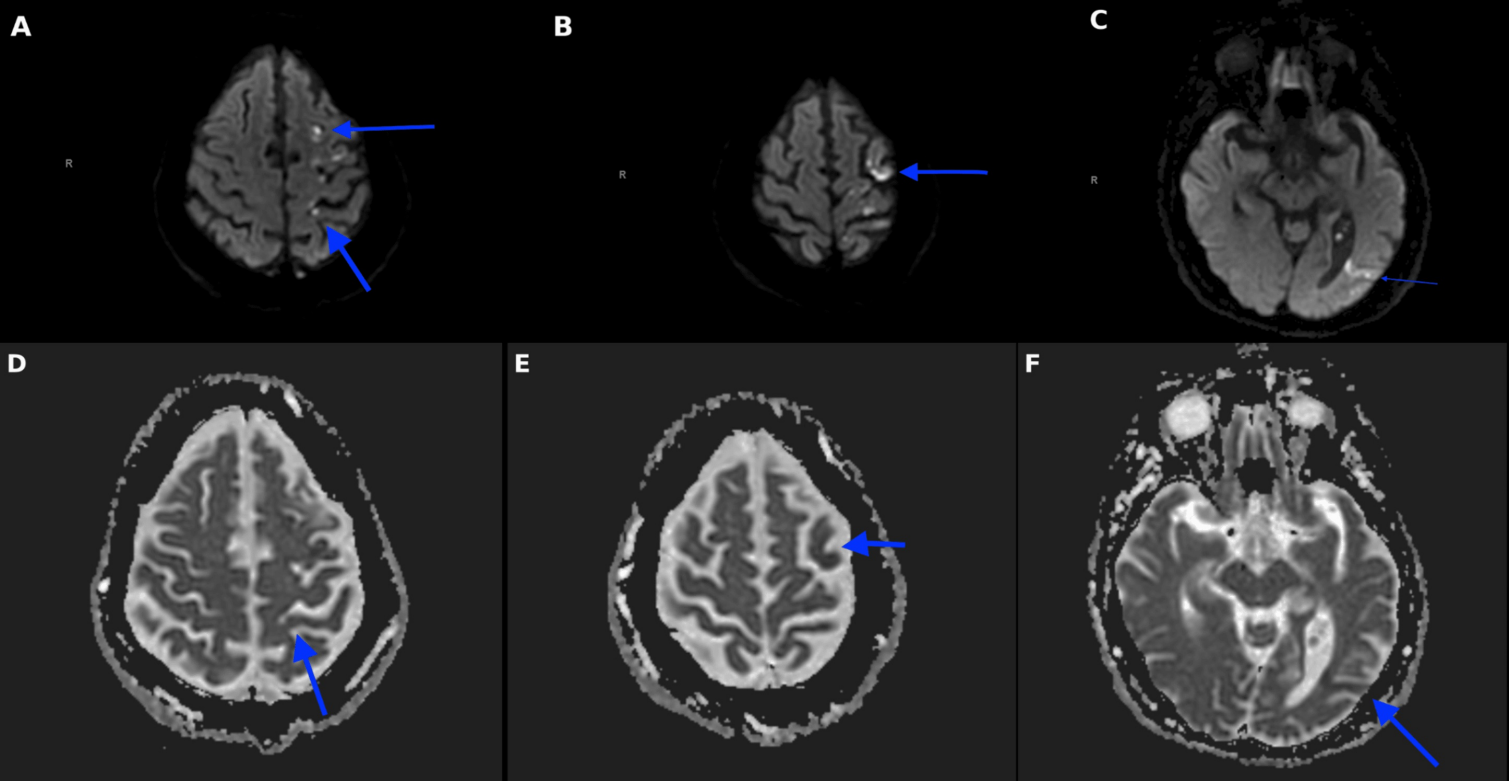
Case 1: (A-F) Numerous areas of restricted diffusion involving the left frontal lobe, parietal lobe, and left MCA/PCA watershed territory with an ADC correlate consistent with an infarct (A-C) Axial MRI brain diffusion weighted imaging (DWI); (D-F) Axial MRI brain apparent diffusion coefficient (ADC) MCA: middle cerebral artery; PCA: posterior cerebral artery
